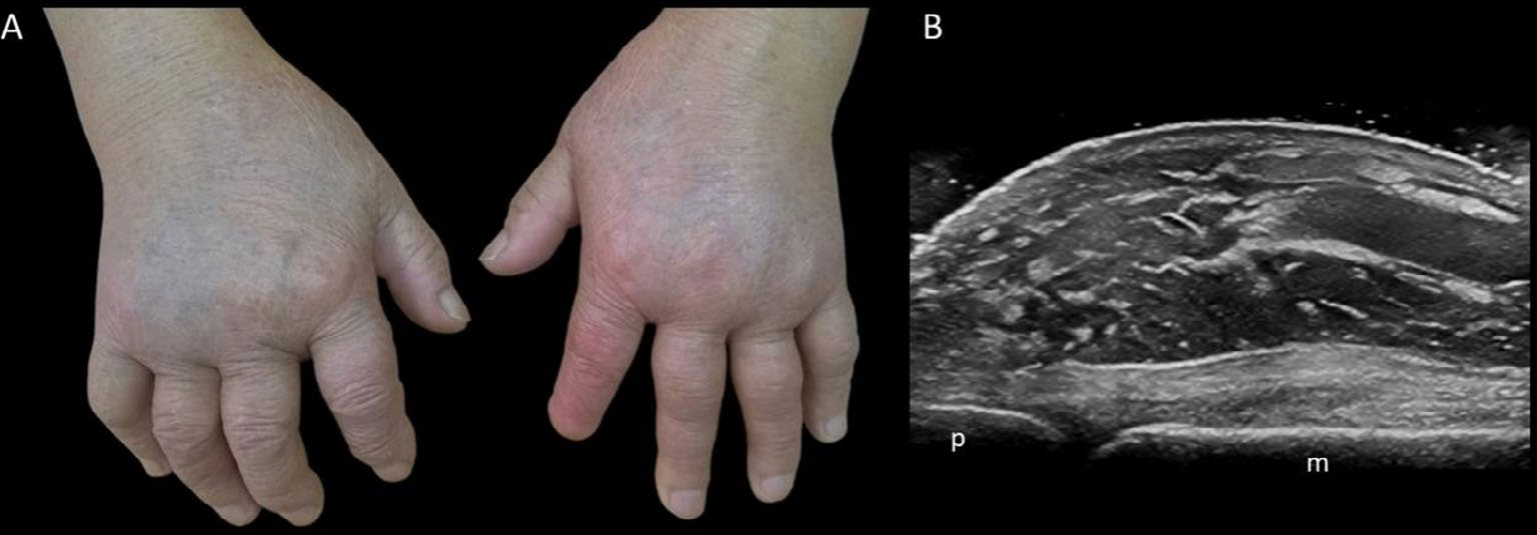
Remitting seronegative symmetrical synovitis with pitting edema (RS3PE) syndrome. An 85‐year‐old woman with recent onset of inflammatory pain associated with swelling of the hands and feet. (A) Clinical image showing the presence of bilateral pitting edema on the dorsum of the hands. (B) Ultrasound image (linear probe, 6–18 MHz) at the level of the third metacarpophalangeal joint of the right hand, demonstrating subcutaneous soft tissue swelling in the absence of clear signs of joint inflammation. m, metacarpal bone; p, base of the proximal phalanx.
